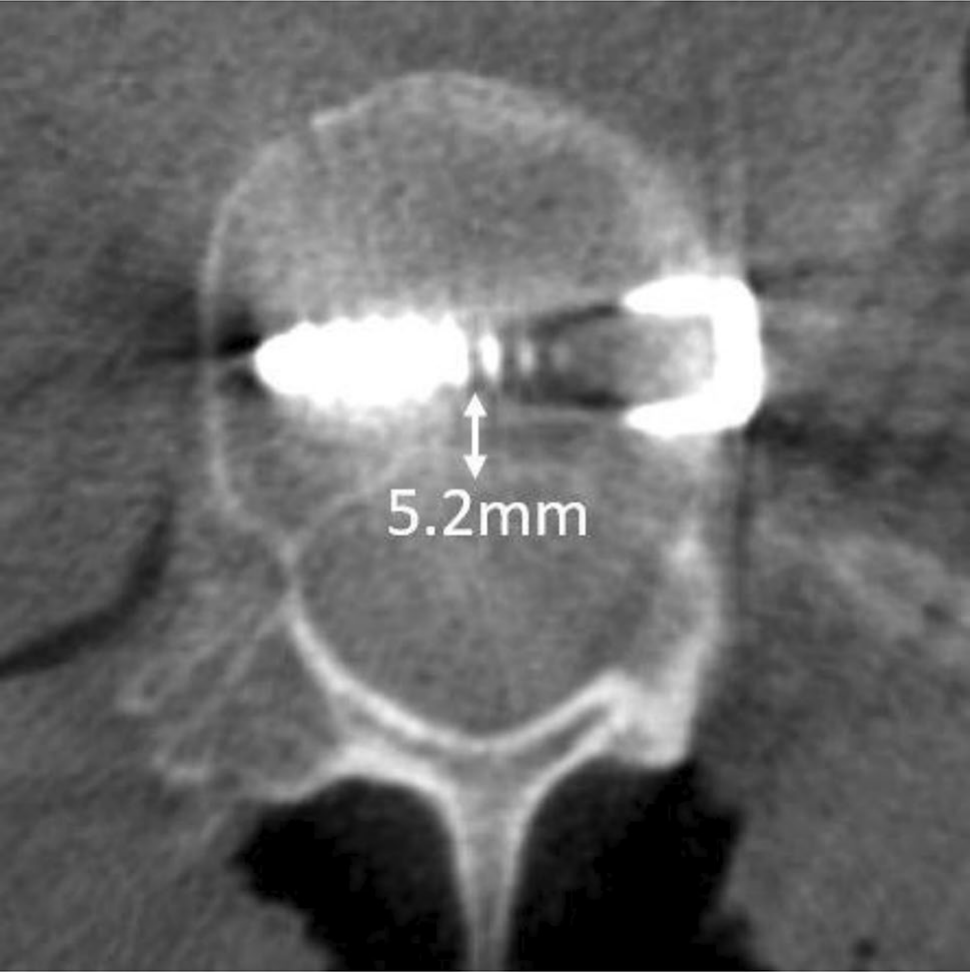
Axial CT image showing measurement of the closest distance of a screw to the spinal canal
